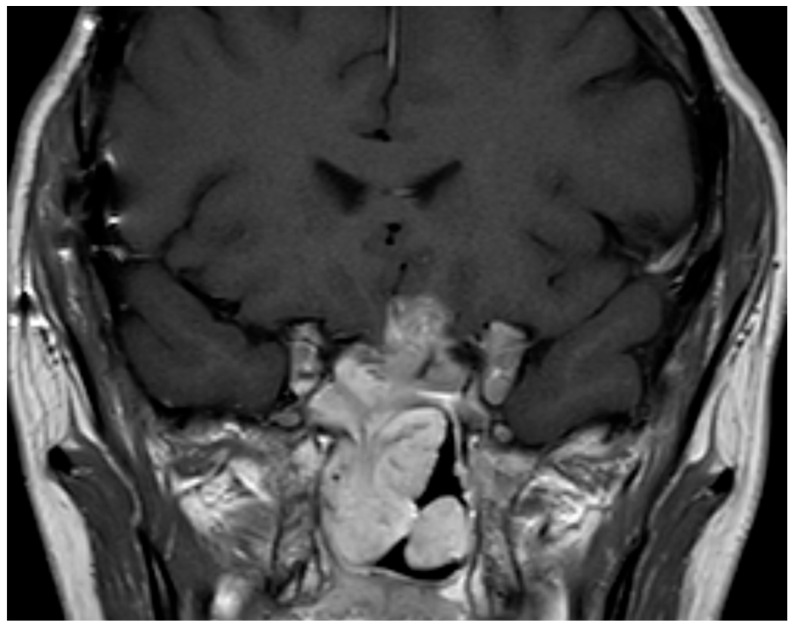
Coronal pituitary magnetic resonance image with T1-weighted contrast-enhanced T1 sequence showing the image of a persistent aggressive silent corticotropinoma after 4 surgeries plus radiotherapy.
